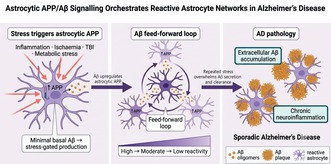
AbbreviationsADAlzheimer's diseaseADAM‐10a disintegrin and metalloproteinase domain‐containing protein 10AICDAPP intracellular domainAPH‐1anterior pharynx‐defective 1APLP1amyloid precursor‐like protein 1APLP2amyloid precursor‐like protein 2ApoEapolipoprotein EAPPamyloid precursor proteinAQP4aquaporin‐4Aβamyloid‐β peptidesBACE1β‐site APP cleaving enzyme 1BACE2β‐site APP cleaving enzyme 2cAMPcyclic adenosine monophosphateCNScentral nervous systemCOX2cyclooxygenase‐2CSFcerebrospinal fluidEAAT1excitatory amino acid transporter 1ERKextracellular signal‐regulated kinaseFDGfluorodeoxyglucoseFOXO3forkhead box O3GFAPglial fibrillary acidic proteinHIF‐1hypoxia‐inducible factor 1IDEinsulin‐degrading enzymeIFNγinterferon gammaIGFinsulin‐like growth factorIL‐10interleukin‐10IL‐12interleukin‐12IL‐18interleukin‐18IL‐1α/βinterleukin‐1 alpha/betaIL‐6interleukin‐6IL‐8interleukin‐8iNOSinducible nitric oxide synthaseJAKjanus kinaseJNKc‐Jun N‐terminal kinaseKPIKunitz‐type protease inhibitor domainLDLRlow‐density lipoprotein receptorLPSlipopolysaccharideLRP1lipoprotein receptor‐related protein 1MCP‐1monocyte chemoattractant protein‐1MMPmatrix metalloproteinaseNADPHnicotinamide adenine dinucleotide phosphateNF‐κBnuclear factor kappa‐light‐chain‐enhancer of activated B cellsNLRP3NOD‐like receptor protein 3NOnitric oxideOX2MRC OX2 antigenPEN‐2presenilin enhancer 2PETpositron emission tomographyPGE2prostaglandin E2PKAprotein kinase APKCprotein kinase CPSEN1presenilin 1RNAribonucleic acidROSreactive oxygen speciessAPPαsecreted α‐cleaved APP fragmentsAPPβsecreted β‐cleaved APP fragmentSTAT3signal transducer and activator of transcription 3TBItraumatic brain injuryTDP‐43TAR DNA‐binding protein 43TFEBtranscription factor EBTGFβtransforming growth factor betaTLRtoll‐like receptorTNFα/βtumor necrosis factor alpha/betaTREM2triggering receptor expressed on myeloid cells 2YKL‐40chitinase‐3‐like protein 1
